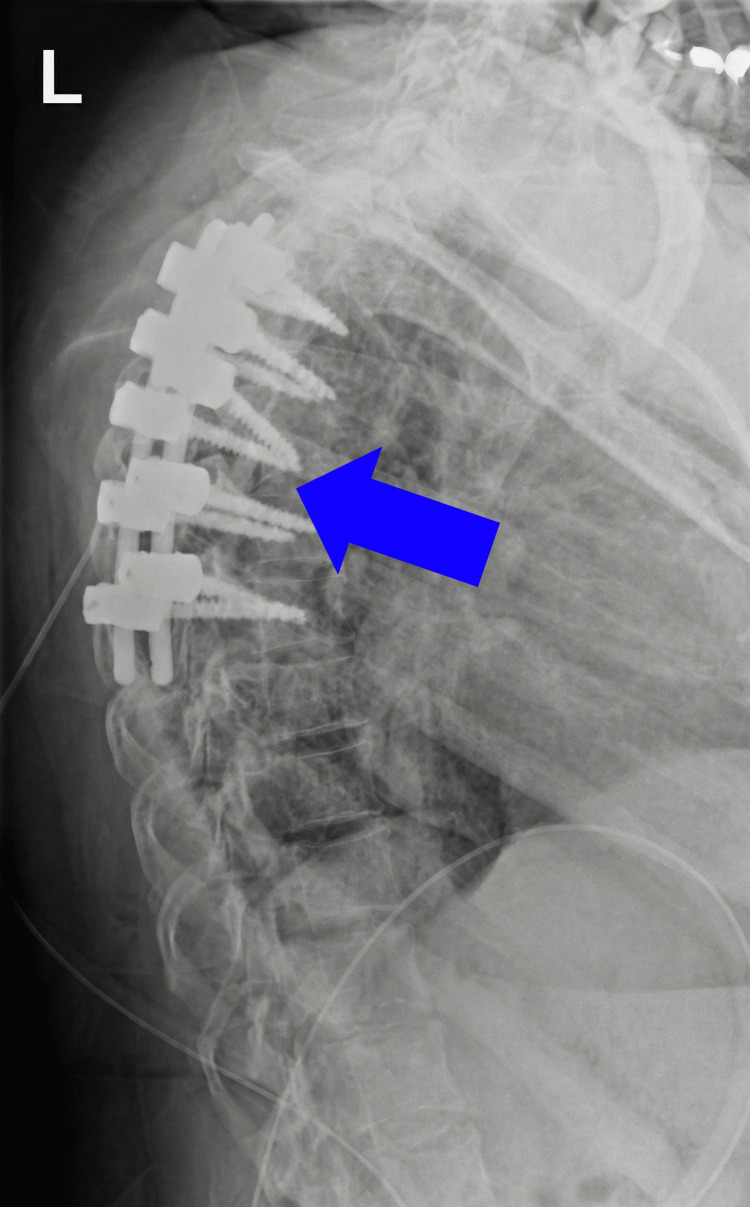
Postoperative lateral radiograph of the thoracic spine
